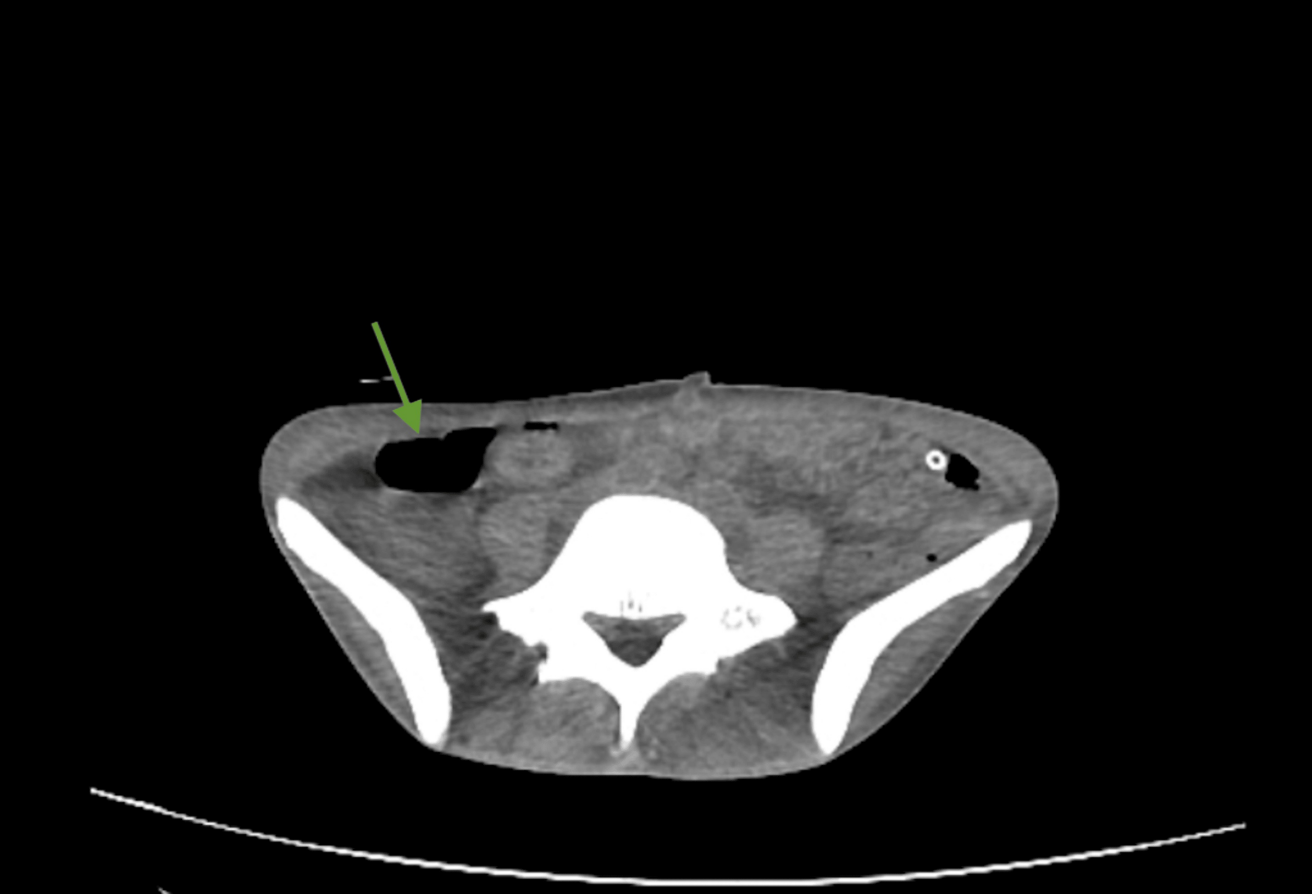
Transverse plane of the pelvis on a contrast enhanced CT showing the fluid levels of the ascites Green arrow: fluid levels of the ascites
